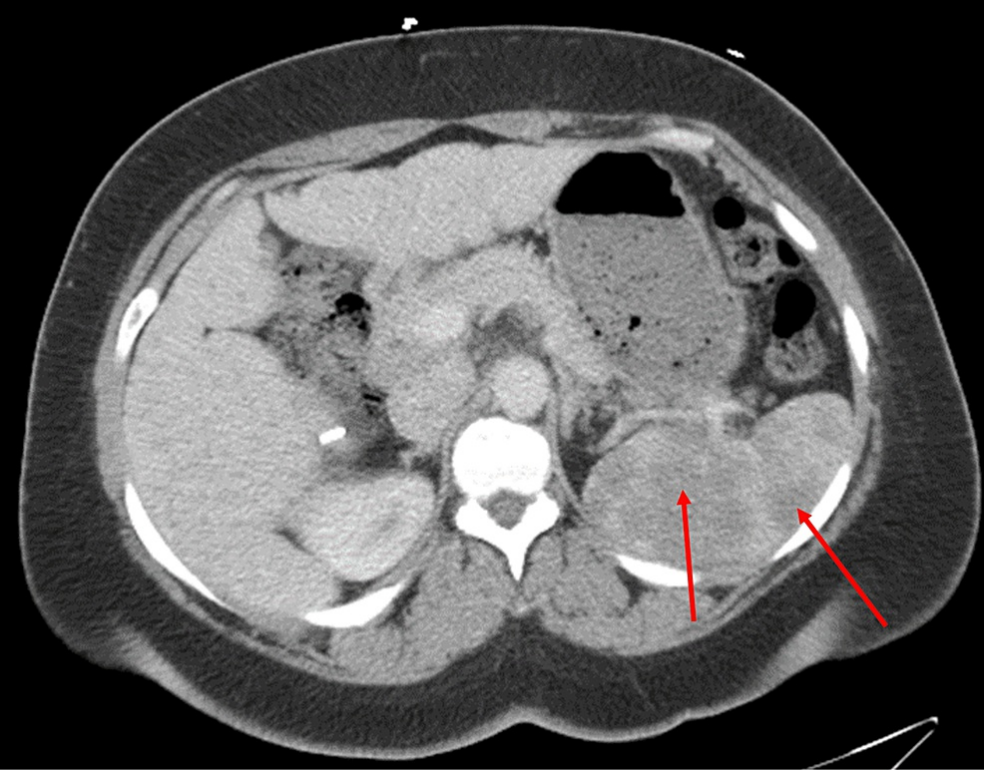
Computed tomography angiography of the abdomen/pelvis showing multiple non-specific hypoattenuating lesions throughout the spleen and heterogeneous liver with nodular morphology.
